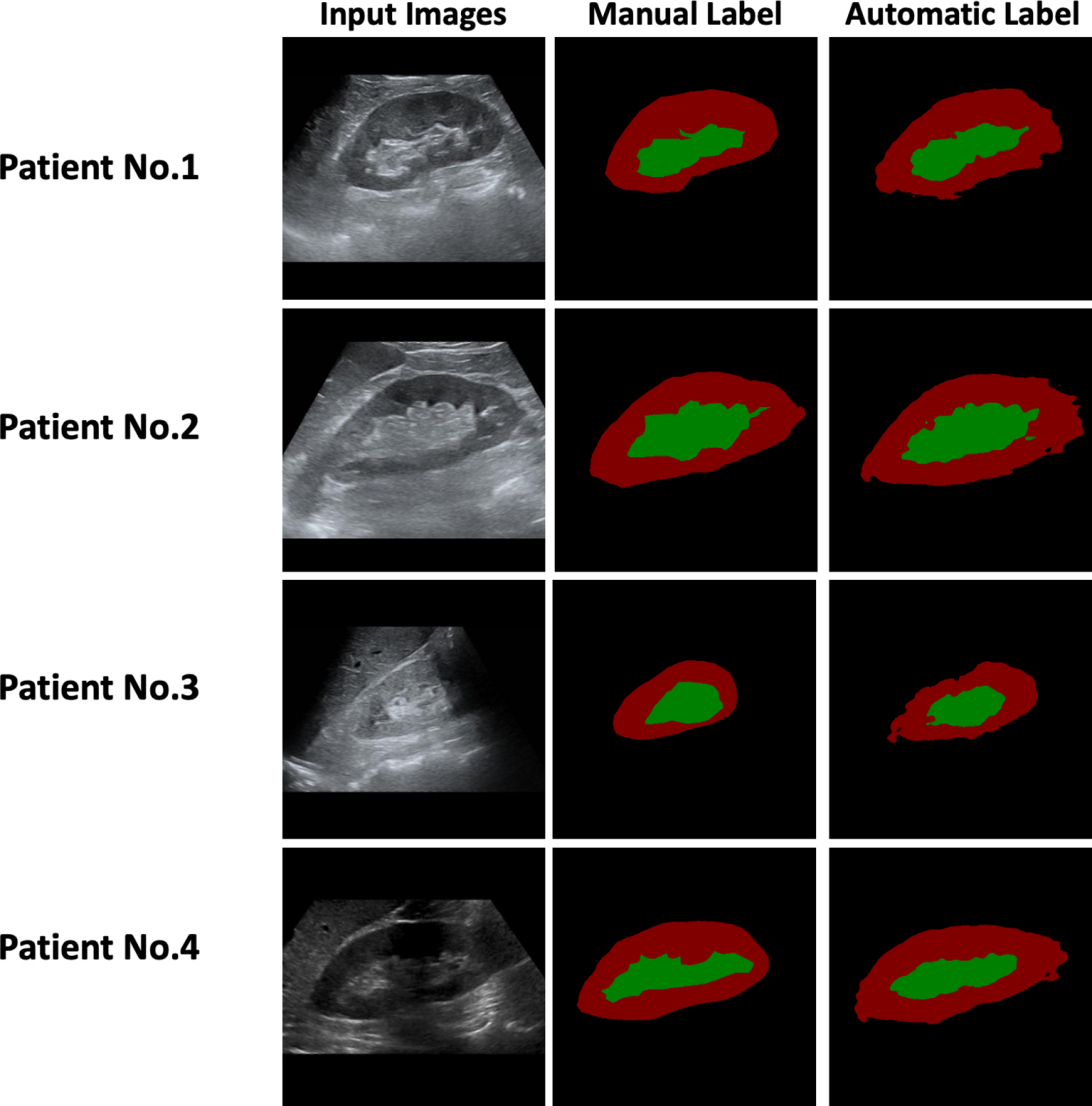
Manual and Automatic Segmentation using Ultrasound Images of the Patients.
